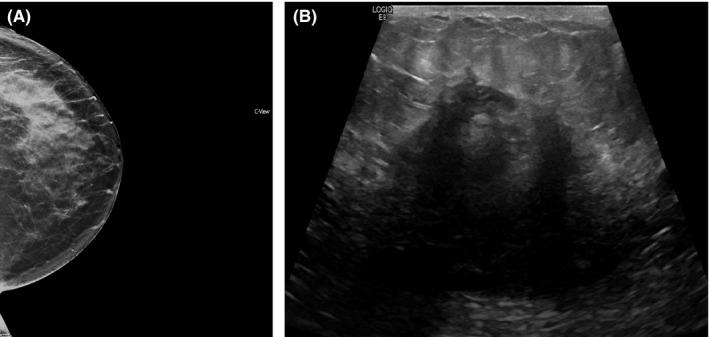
Left breast mammogram revealing asymmetry (A) and ultrasound showing an irregular mass with shadowing (B)
